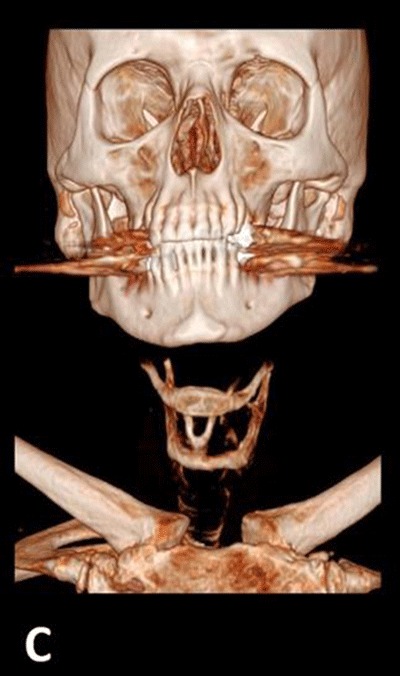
Three-dimensional (3D) volume rendering image of the neck CT-scan showing tilting of the hyoid bone and thyroid cartilage.
